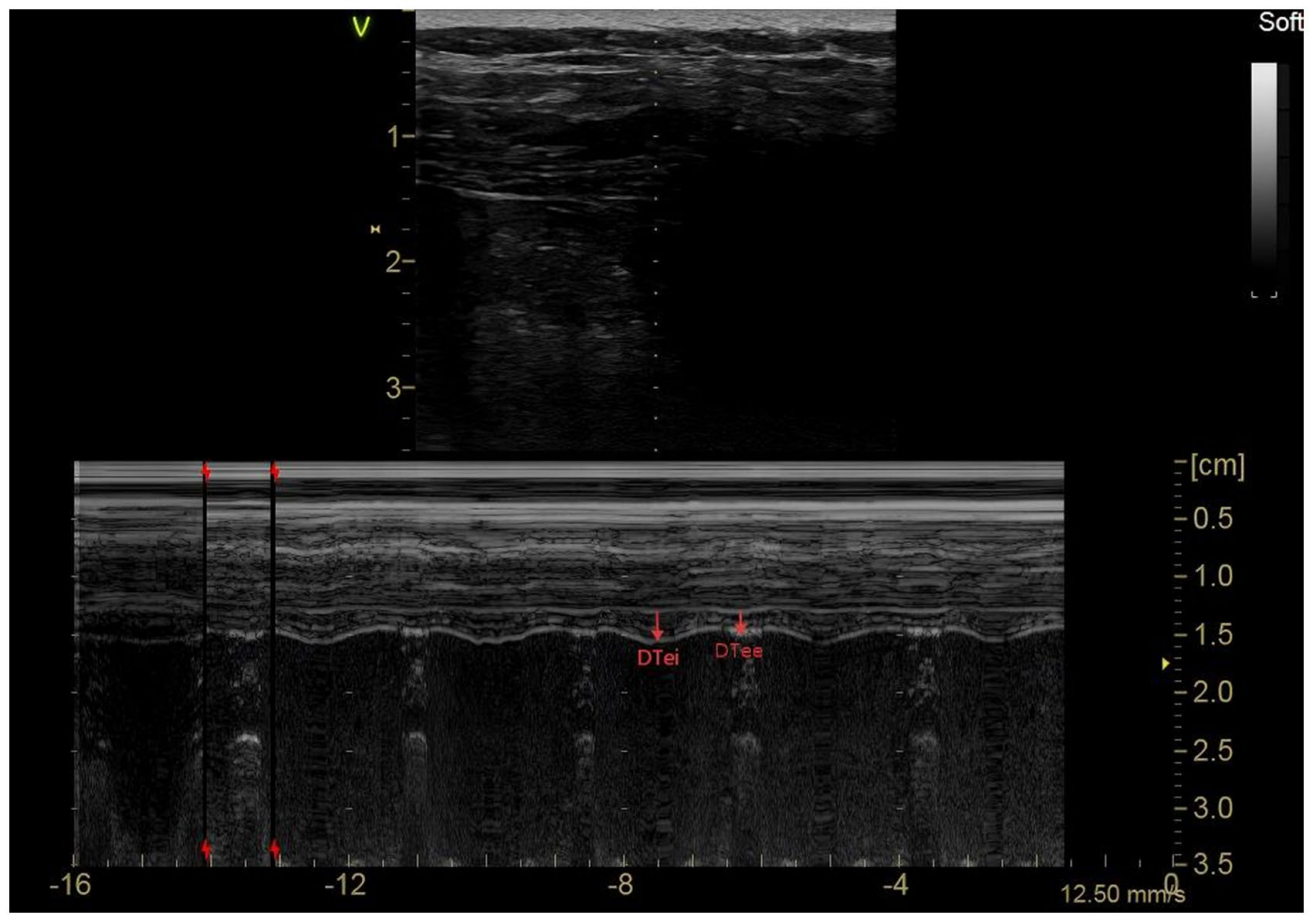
Diaphragm ultrasonography 2: DTei and DTee.
